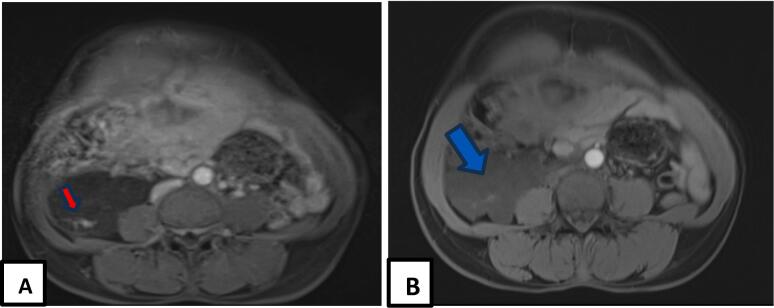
Axial sections on T1-weighted pelvic MRI without (A) and with (B) contrast injection showing the septa with a discrete T1 hypersignal (red arrow) and that the mass moderately increased after injection of the contrast medium (blue arrow). (For interpretation of the references to colour in this figure legend, the reader is referred to the web version of this article.)
